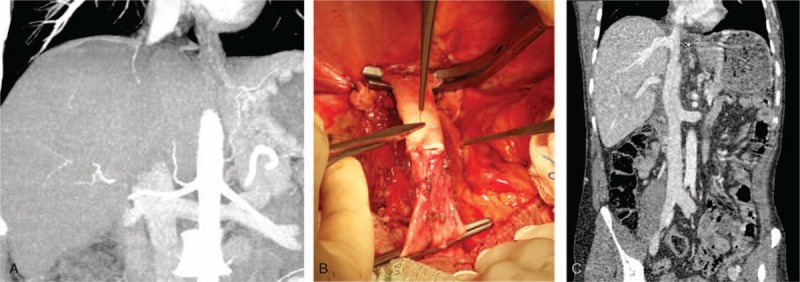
Coronal CT image showing complete occlusion of a retrohepatic VCI (A). After the occluded segment of the VCI was excised, venous continuity was established with an aortic vascular graft (B). Coronal CT image showing the posttransplant venous drainage (C). CT = computed tomography, VCI = vena cava inferior.
